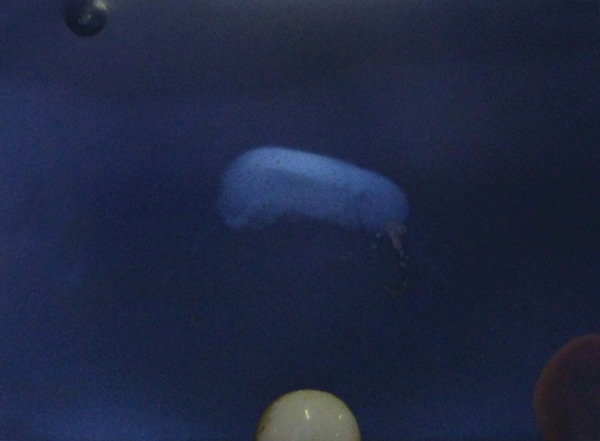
Radiograph of lip confirmed presence of tooth fragment in lower lip
